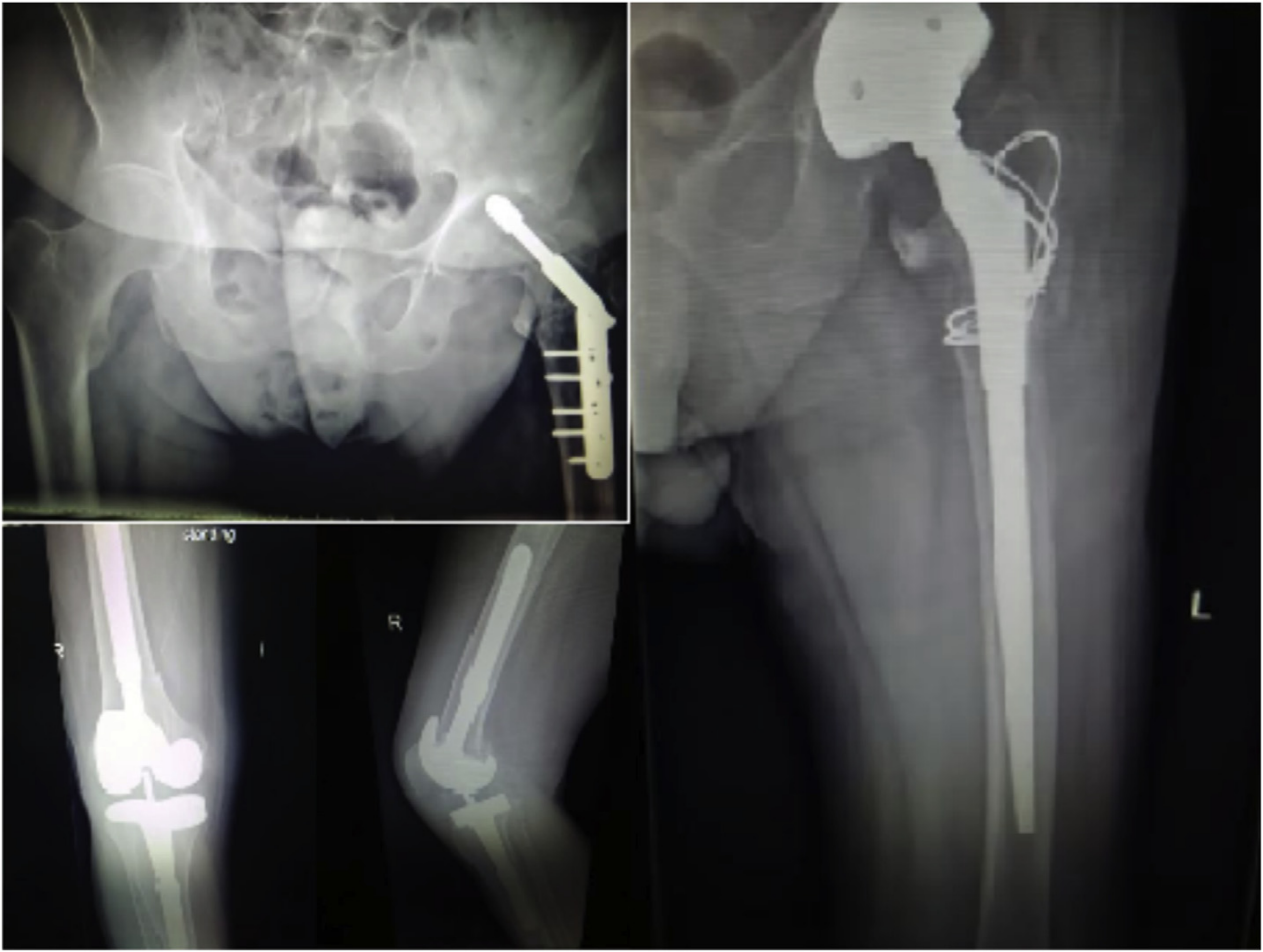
X-ray images showing the dynamic hip screw cut-out from the left hip and the totally asymptomatic right knee prosthesis and postoperative left hip image.
